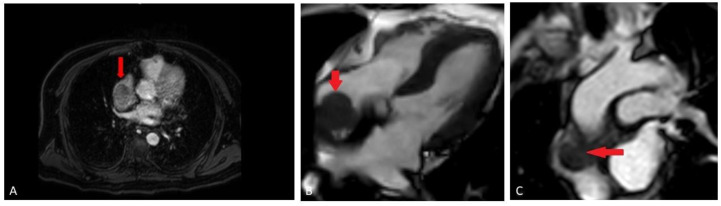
(A) Cardiac MRI early post-contrast mDixon sequence (fat suppressed gradient echo sequence), demonstrates enhancing component of the mass in the distal left Superior Vena Cava (SVC); (B) cardiac MRI pre-contrast balanced turbo field echo (BTFE) sequence, demonstrating adherent mass (red arrow) along the right atrial free wall; (C) cardiac MRI early post-contrast perfusion sequence (T1 weighted, gradient-echo), demonstrating relatively homogeneous enhancement of the mass (red arrow).
